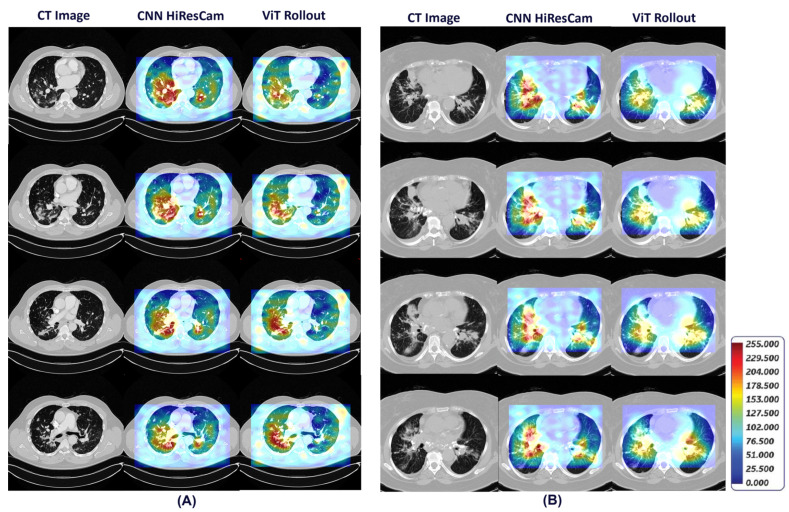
HiResCAM and ViT Attention Rollout visual explanations that highlight the regions of interest on CT scan associated with diagnosis of pulmonary sarcoidosis (A) and lung cancer (B).
